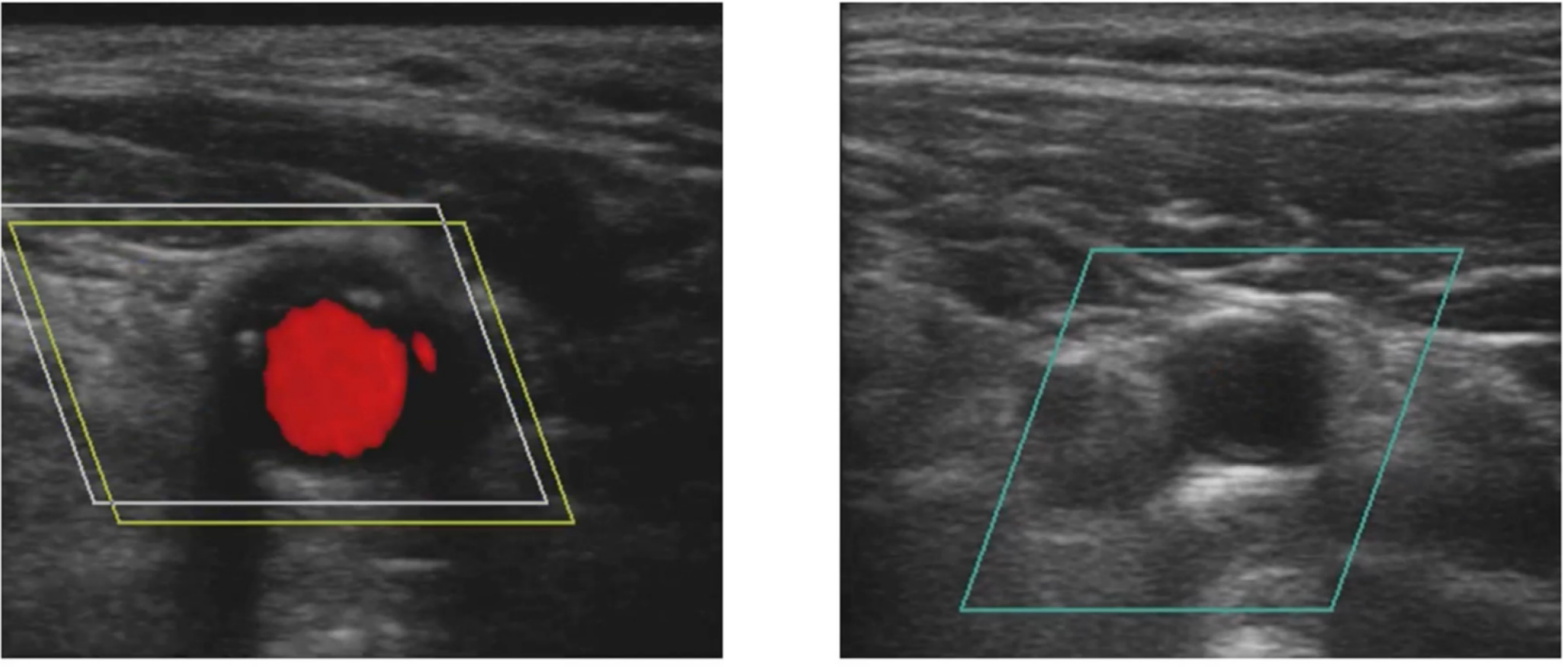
The figure shows an example screenshot from the six-minute introductory video to the study, which was presented to each study participant before the examination was carried out. It shows an ultrasound examination of the CCA in Color Doppler: on the left with a pulsating CCA and on the right without pulsation of the CCA (with clamped aorta).
